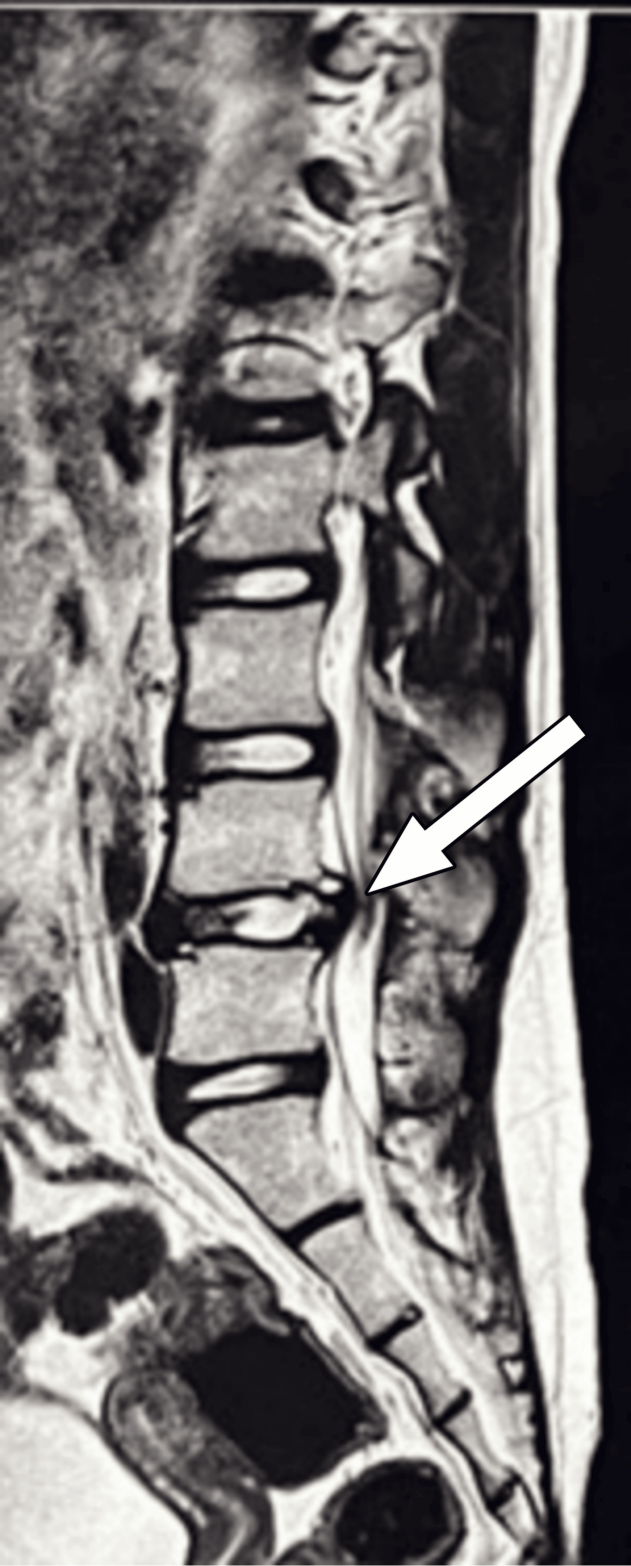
Sagittal section of the MRI of the LS spine The sagittal section of the MRI of the lumbosacral spine showing partial disc desiccation with diffuse disc bulge at the L4-L5 level (white arrow). MRI: magnetic resonance imaging, LS: lumbosacral
